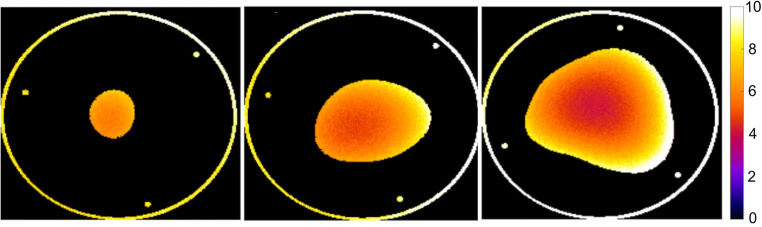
Average absorbed dose in mGy as a function of the X-ray tube current
